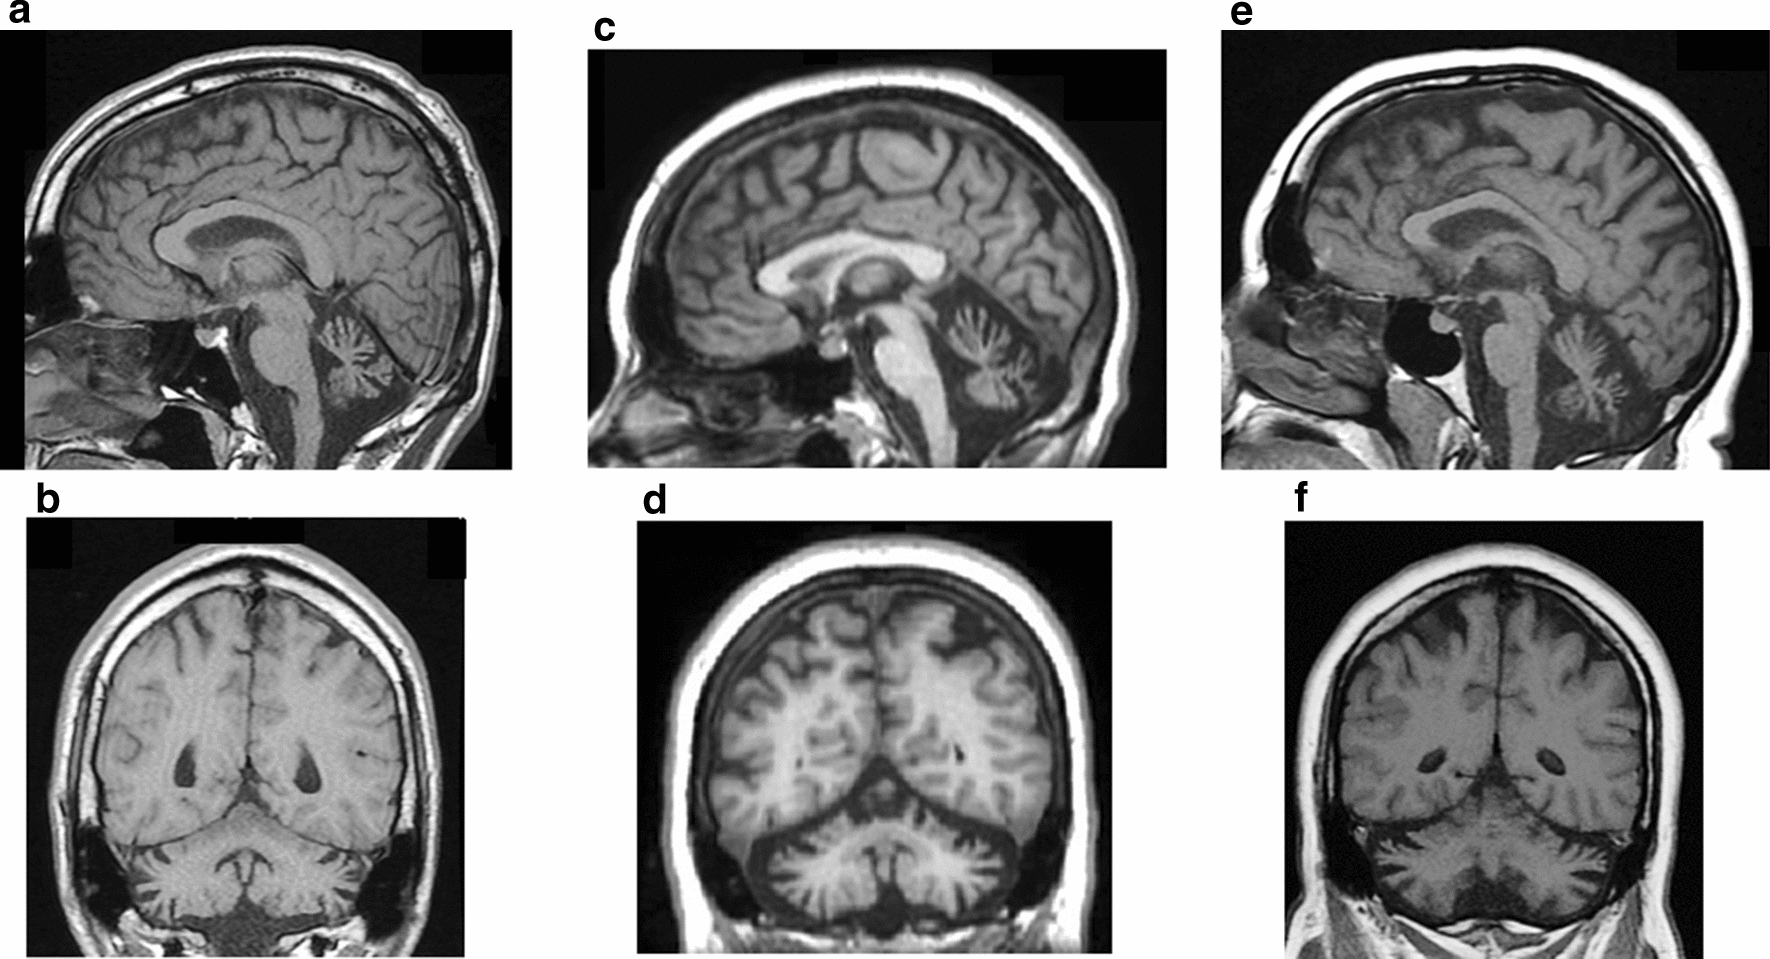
Brain MRI scans of *SYNE1* ataxia patients demonstrated moderate cerebellar atrophy in all subjects and mild cortical atrophy in AT-05 and AT-06 patients. a, b: AT-04 patient; c, d: AT-05 patient; e, f: AT-06 patient; a, c, e: sagittal T1 weighted scans; b, d, f: coronal T1-weighted scans
